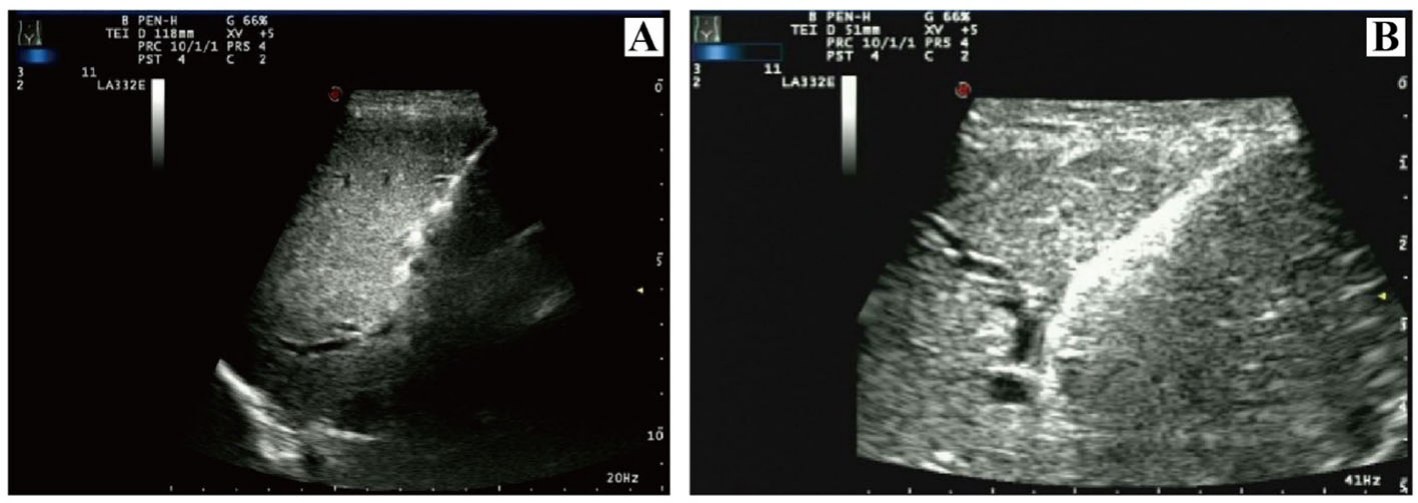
Ultrasound imaging of PTCD performed to improve jaundice and biliary tract infection. (A) initial catheter placement; (B) catheter replacement. PTCD, percutaneous transhepatic cholangial drainage.
